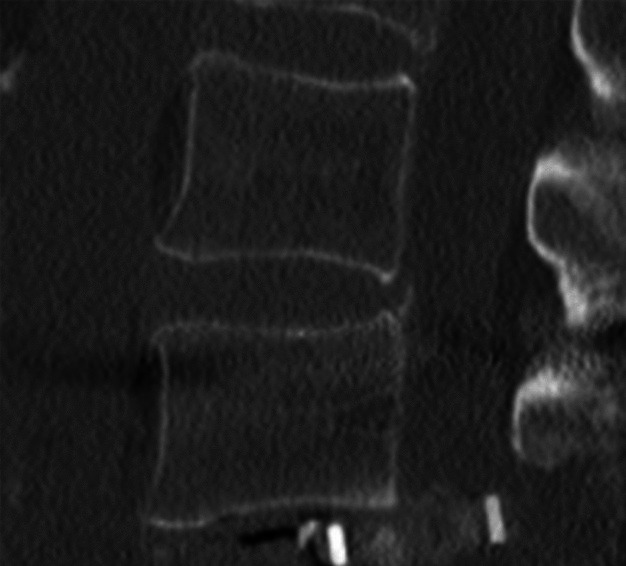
Computed tomography of migrated cage for case 3 Source: Moisi M, Page J, Paulson D, Oskouian RJ (2015) Technical Note – Lateral Approach to the Lumbar Spine for the Removal of Interbody Cages. Cureus 7(5): e268. 10.7759/cureus.268
